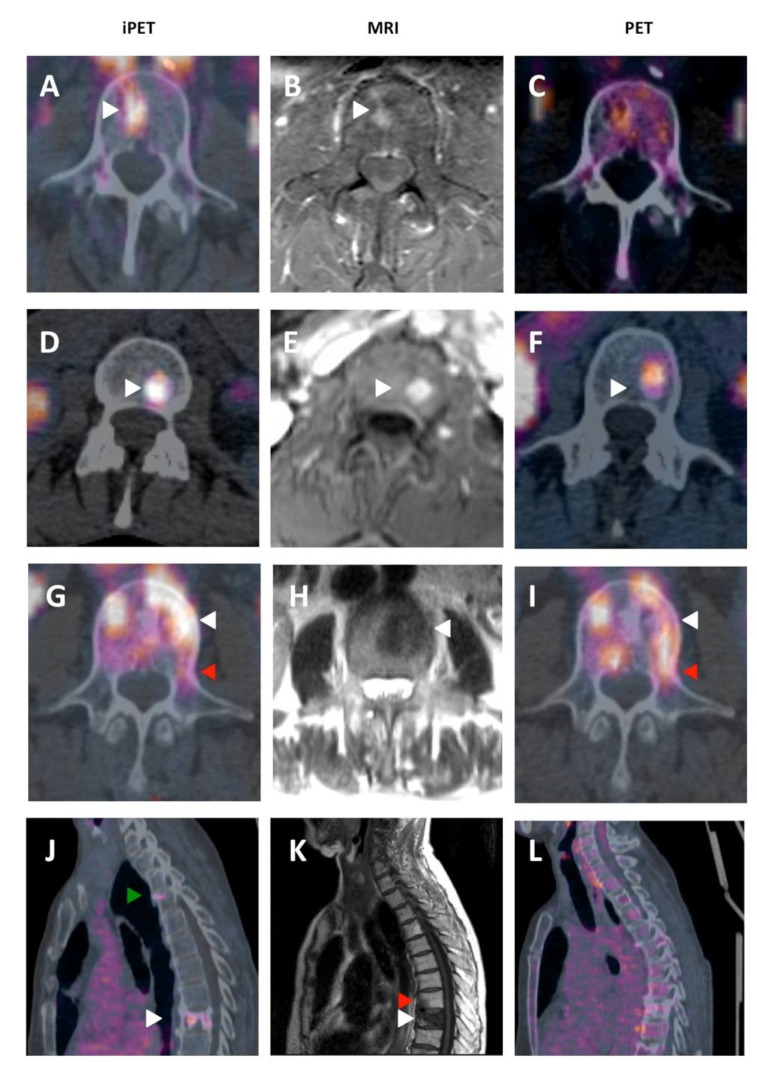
Representative cases of iPET/CT, MRI and PET/CT imaging in VM. (A–C) 51-year-old female with metastatic breast cancer: L4 spinal metastasis. (A) Axial iPET/CT: increased tracer uptake in vertebral body (white arrow); (B) axial MRI with T1 weighting and injection of gadolinium contrast: hypersignal lesion in vertebral body (white arrow). (C) Axial PET-CT ^18^FDG: background tracer uptake, no visible lesion. (D–F) 46-year-old female with metastatic medullary thyroid carcinoma: L3 spinal metastasis. (D) Axial iPET/CT: increased tracer uptake in vertebral body (white arrow). (E) Axial MRI with T1 weighting and injection of gadolinium contrast: hypersignal lesion in vertebral body (white arrow). (F) Axial PET-CT ^18^F-DOPA: increased tracer uptake in vertebral body (white arrow). (G–I) 47-year-old female with metastatic breast cancer: L3 spinal metastasis. (G) Axial iPET/CT: increased tracer uptake in vertebral body (white arrow) and extension in left pedicle (red arrow). Tumor volume diffusely involves the vertebral body and the left pedicle in iPET. The corresponding Clinical Target Volume (CTV) would thus include, according to the International Spine Radiosurgery Consortium (ISRC), the entire vertebral body, the left pedicle, and ipsilateral transverse process and lamina. (H) Axial MRI with T2 weighting: hypersignal lesion in vertebral body (white arrow) without extension in left pedicle. This MRI would suggest a CTV that includes the entire vertebral body and the ipsilateral pedicle. (I) Axial PET-CT ^18^FDG: increased tracer uptake in vertebral body (white arrow) and extension in left pedicle (red arrow). (J–L) 57-year-old female with metastatic breast cancer: T2, T8, T9 spinal metastasis. (J) Sagittal iPET/CT: increased tracer uptake in T2 (green arrow) and T9 (white arrow) vertebral bodies. The lesion in T8 is not visible. (K) Axial MRI with T1 weighting: hyposignal lesion in T8 (red arrow) and T9 (white arrow) vertebral bodies. The lesion in T2 is not visible. (L) Sagittal PET-CT ^18^FDG: background tracer uptake, no visible lesion.
